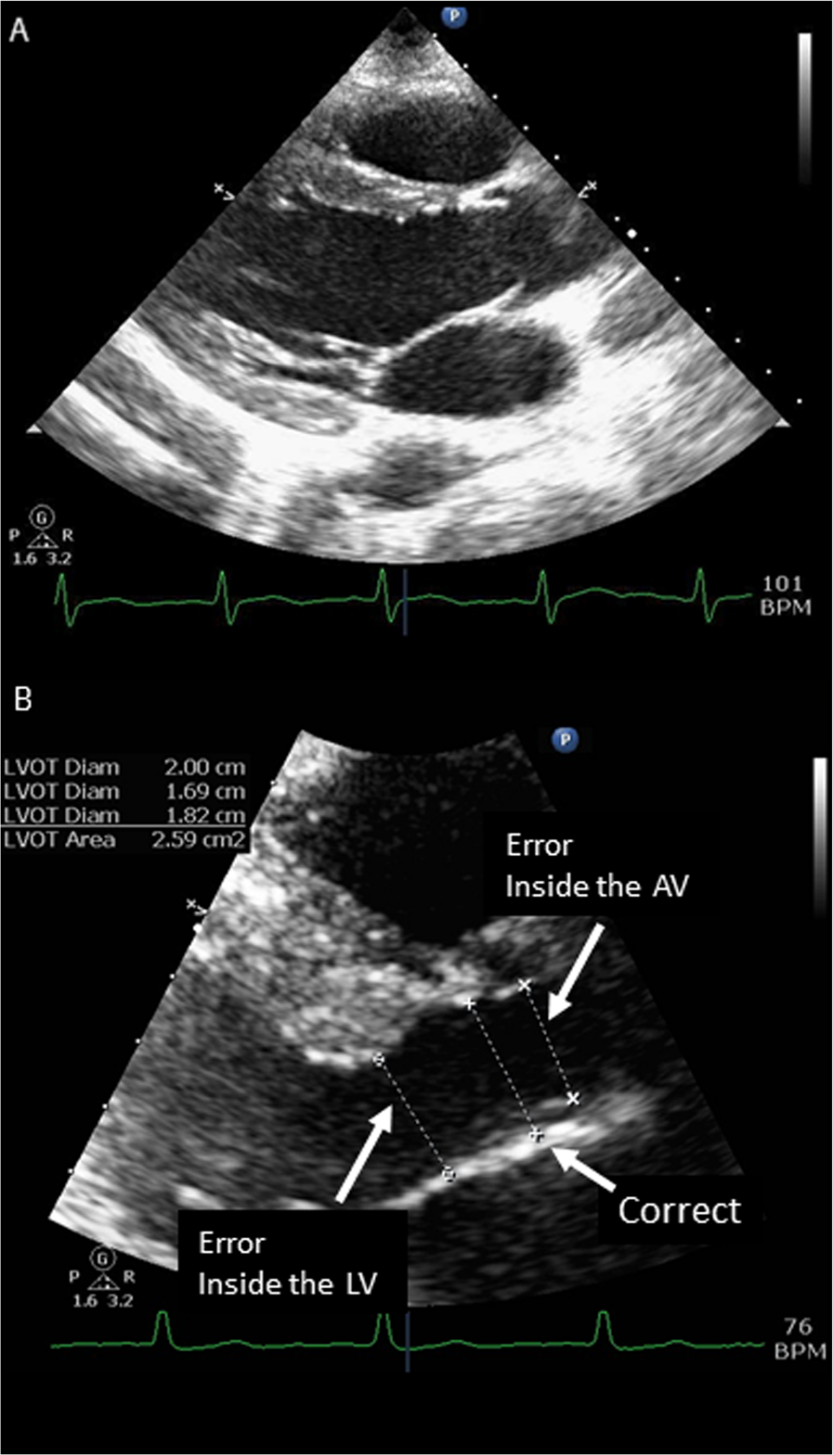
Panel a: Example of ideal image obtained in parasternal long at initiation of systole, Panel b:ss Magnification of LVOT and examples of common errors in measurement
